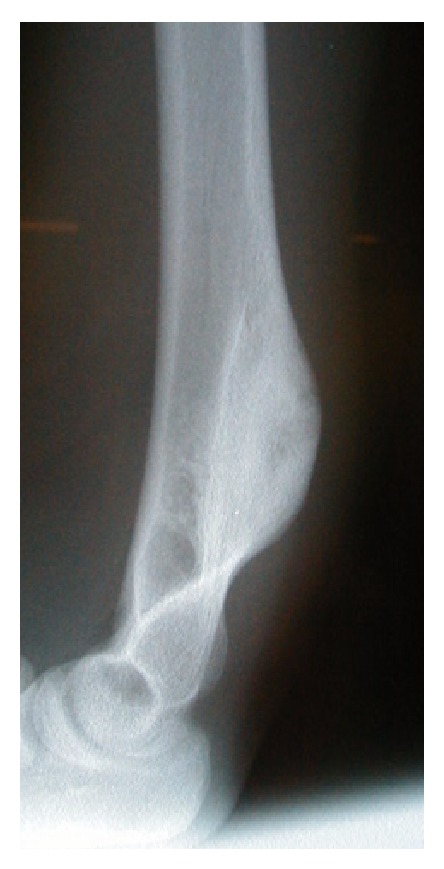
Lateral X-ray of the distal right humerus showing a dense bony protuberance arising from the distal aspect of the humerus on the posterior surface without periosteal reaction.
